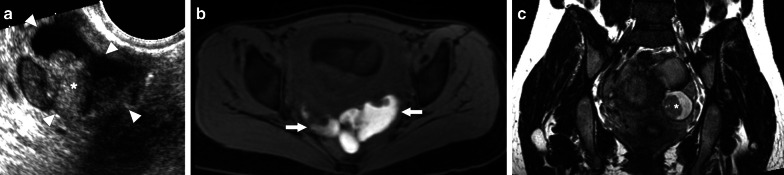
Decidualized endometriomas. A 24-year-old pregnant patient with previous history of menstrual irregularity presenting for routine obstetric US. a US showed a normal intrauterine pregnancy with biometry compatible with 12 weeks and 5 days gestation (not shown). However, there were bilateral (left not shown) adnexal complex cystic lesions (arrowheads) with solid components (asterisk). About 10 days later, axial T1W fat-suppressed (b) and coronal T2W (c) non-contrast pelvic MR images showed bilateral T1 hyperintense endometriomas (arrows) with T2 hypointense nodules (asterisk) in the lesions bilaterally (right not shown). She was managed expectantly. She was induced at 41 weeks and the baby was healthy. Follow-up MRI at 12 and 30 months post-partum (not shown) showed diminished size of the lesions with some complexity remaining on the left. Two years after the first delivery she delivered a second child uneventfully
